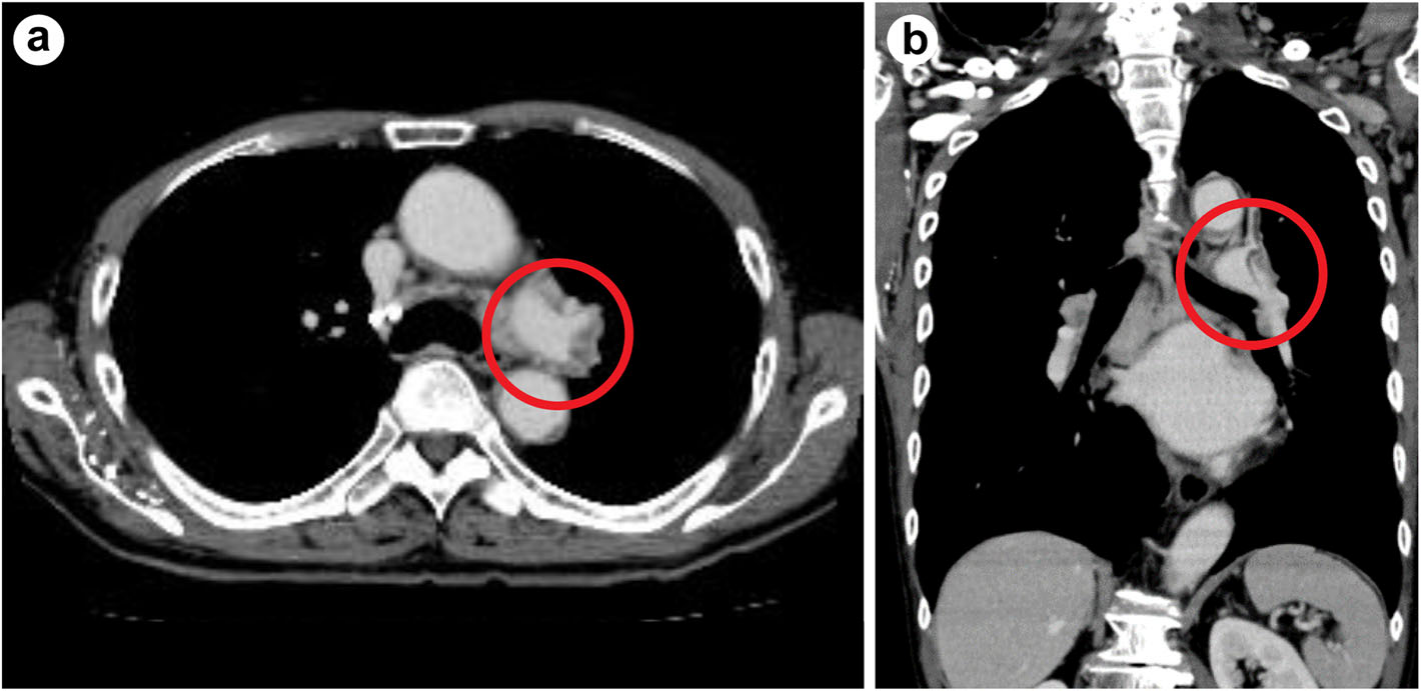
Thoracoabdominal contrast-enhanced CT on Day 14. The CT showed a contrast defect area in the main trunk of the left pulmonary artery
